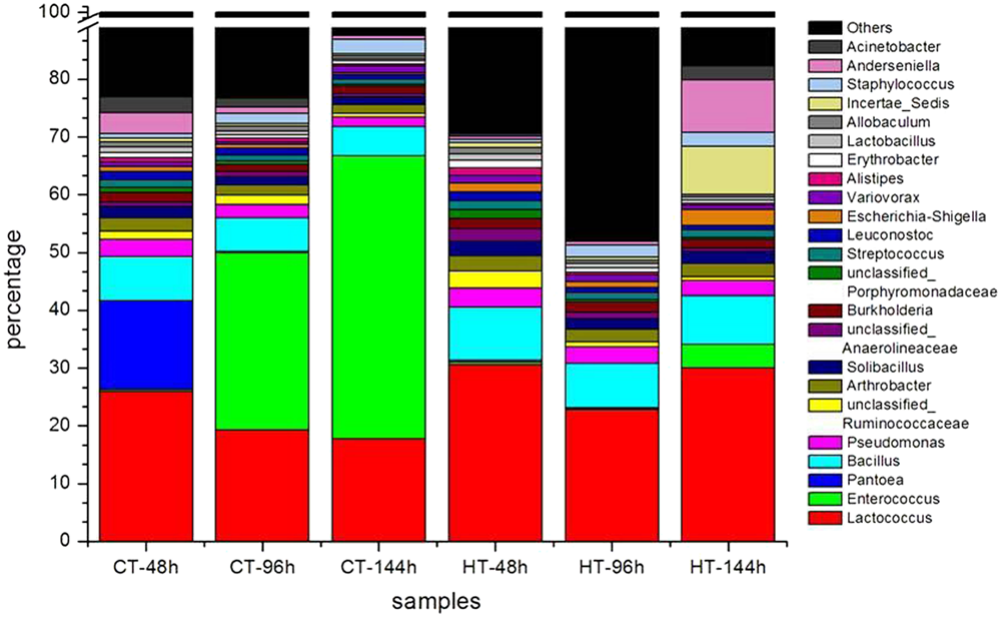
The dominant genera percentage for all samples at each time point. CT/HT-N is sample mentioned in Table 1.
